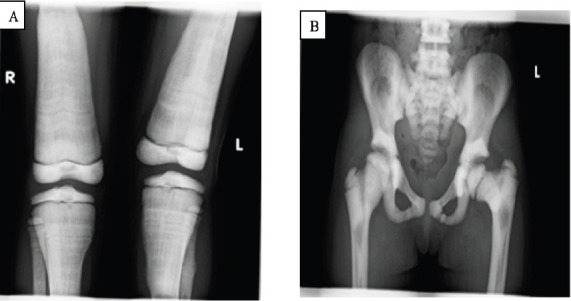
(A) Radiographs of the bilateral knee demonstrating metadiaphyseal widening with vertical and horizontal striations as well as loss of corticomedullary differentiation. (B) Pelvic x-ray demonstrating diffuse sclerosis with some spared normal-density bone indicating a “bone in bone” appearance, and lower lumbar vertebrae showing “Rugger jersey spine” or “sandwich vertebrae”
